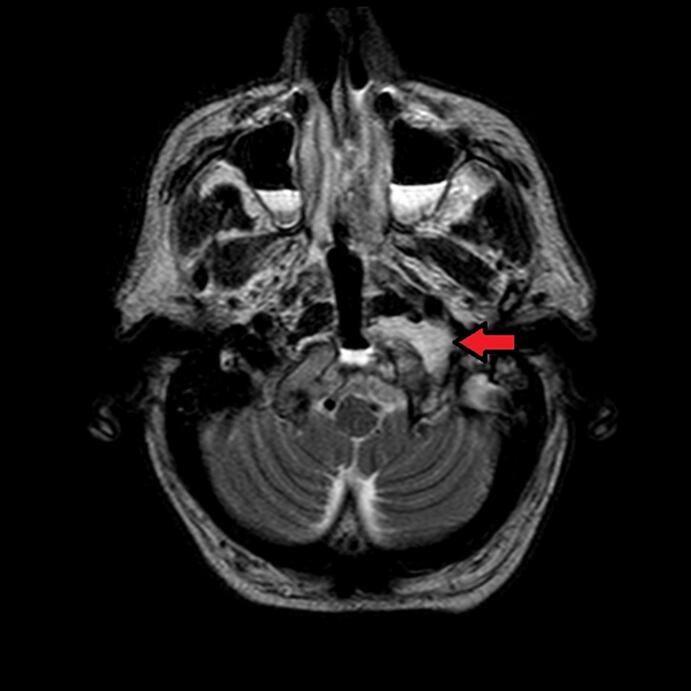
T2-weighted MRI, axial view. Signs of surgical intervention on the clivus are noted. The arrow shows residual tumor. (post-operative MRI).
